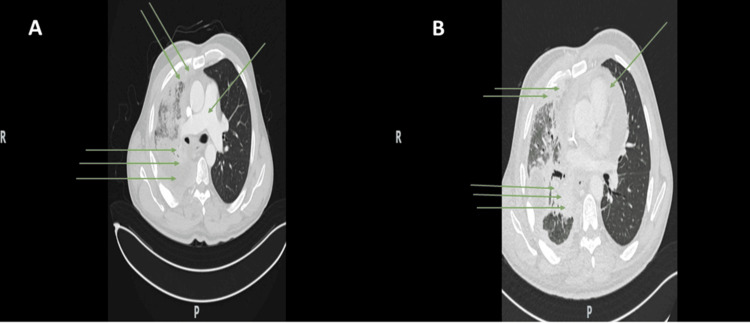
Contrast-enhanced chest computed tomography (CT) Axial contrast-enhanced CT images at the level of the great vessels (A) and upper cardiac chambers (B) demonstrate significant disease progression and malignant cardiac encasement. The mass directly infiltrates the mediastinal pleura and pericardium, becoming inseparable from the adjacent epicardial fat pads. Panel A: The single green arrow identifies the pulmonary artery and its primary branches. Double green arrows highlight the enhancing malignant soft tissue in the right anterior mediastinum. Triple green arrows demonstrate the paravertebral soft tissue involvement adjacent to the thoracic spine, encroaching upon the adjacent right-sided neuroforamina and intercostal musculature. Panel B: The single green arrow points to a moderate pericardial effusion within the "rigid shell." The double green arrows identify the right-sided anterior mediastinal soft tissue. The triple green arrows delineate the diffuse, nodular enhancing pleural thickening along the right lung, as well as the paraspinal mass responsible for the extrinsic compression of the inferior vena cava (IVC) and deformity of the right atrium (RA).
